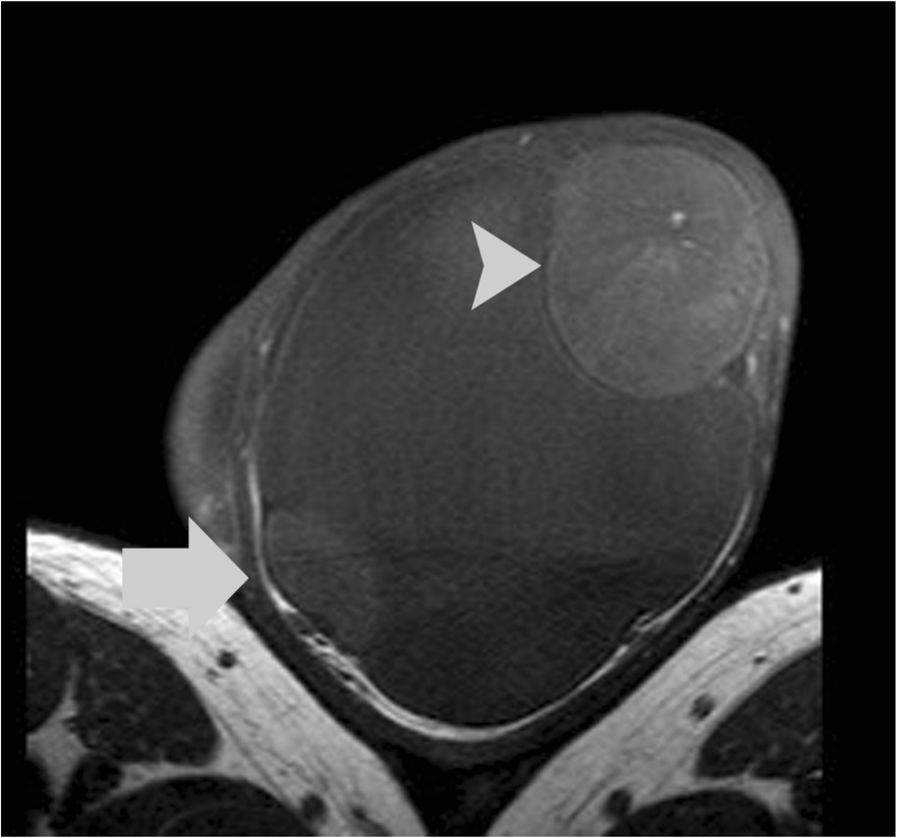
Transverse T1-weighted image shows a well-demarcated left paratesticular mass, lying adjacent to the scrotal wall. The lesion (*arrowhead*) demonstrated mainly similar signal intensity, when compared to the ipsilateral displaced testis (*arrow*). Significant left hydrocele (*long arrow*) was also observed
